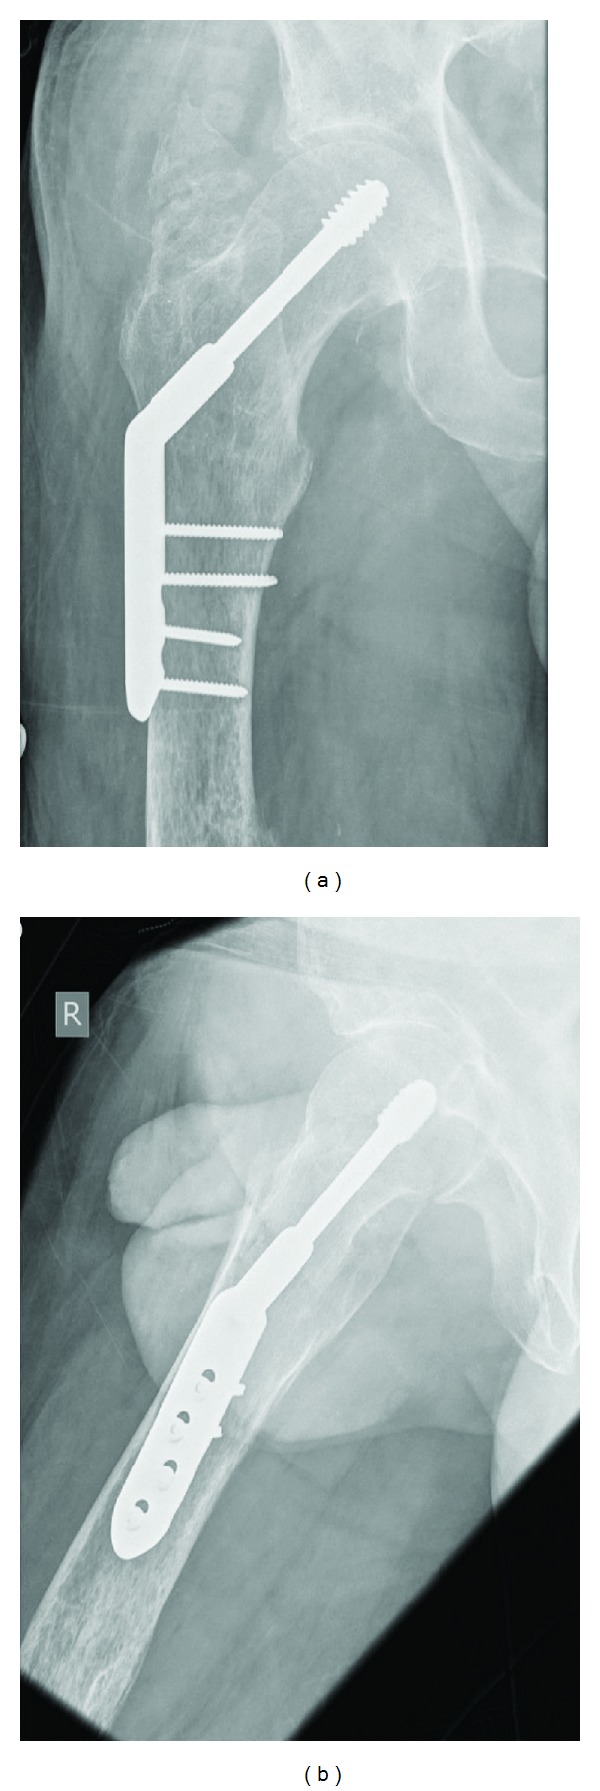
Postoperative radiographs demonstrating satisfactory DHS fixation of the right intertrochanteric hip fracture.
